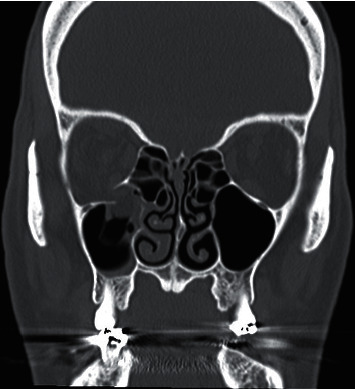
Open globe injury in a 63-year-old man. Axial unenhanced CT image showing concurrent inferior orbital fracture.
